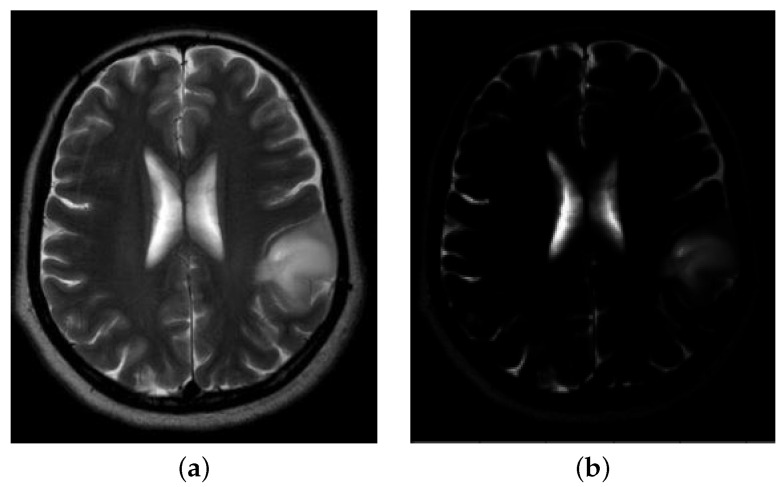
The cerebrospinal fluid appears with high intensity signal for T2-weighted MRIs. (a) image id N22: healthy brain; (b) image id N22 after gamma correction.
